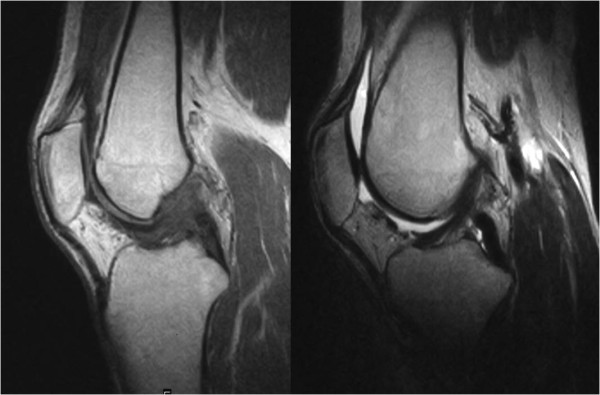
Postoperative MRI (T1WI (left) and T2WI (right)). ACL presented normal signal intensity.
